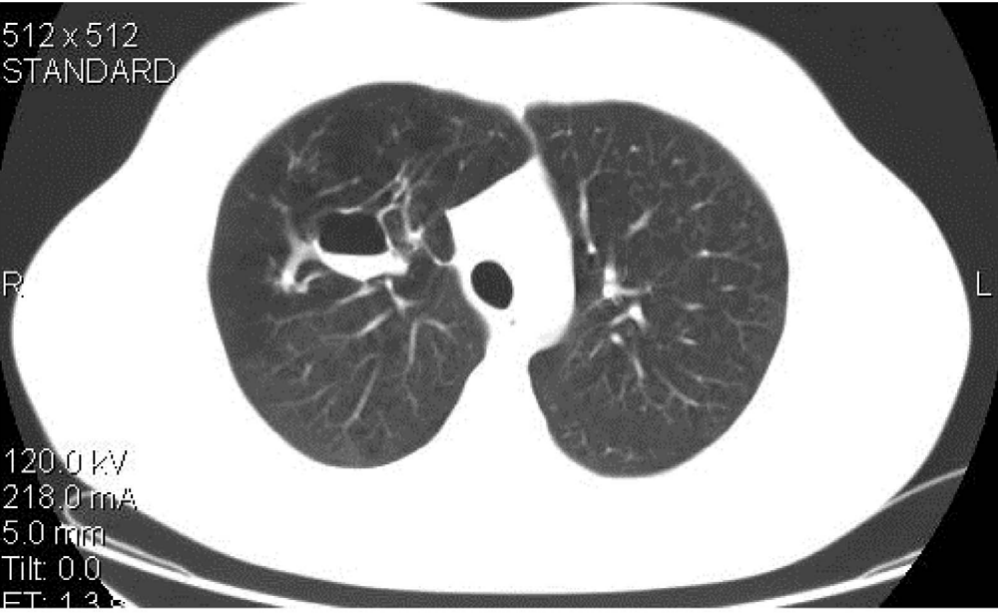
A CT scan of the chest reveals the cavitary lesion with the air-fluid level in it and the distal oligemia and hyperlucency of the lung.
